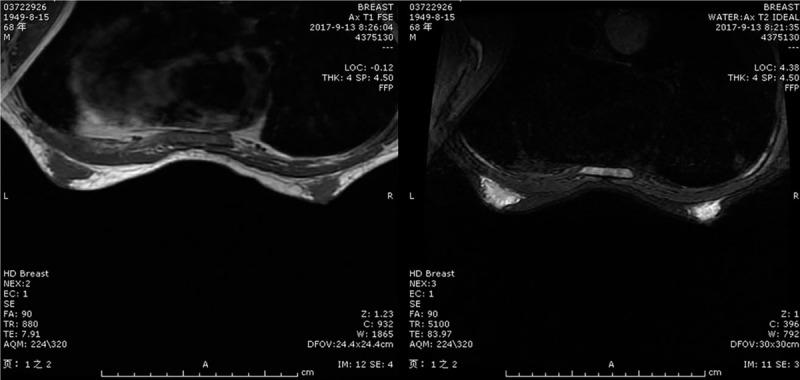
Magnetic resonance imaging T1 and T2 weighted images show developmental mammary gland images.
